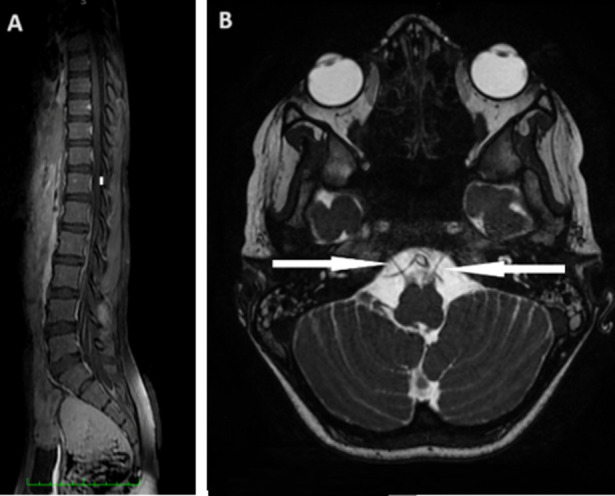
the sagittal T1-weighted MRI with gadolinium enhancement; A) showing enhancement and thickening of the cauda equina roots; the axial FIESTA MRI with gadolinium enhancement; B) demonstrating a right-angled crossing with direct contact between the anterior inferior cerebellar artery and the abducens nerve (VI) bilaterally
